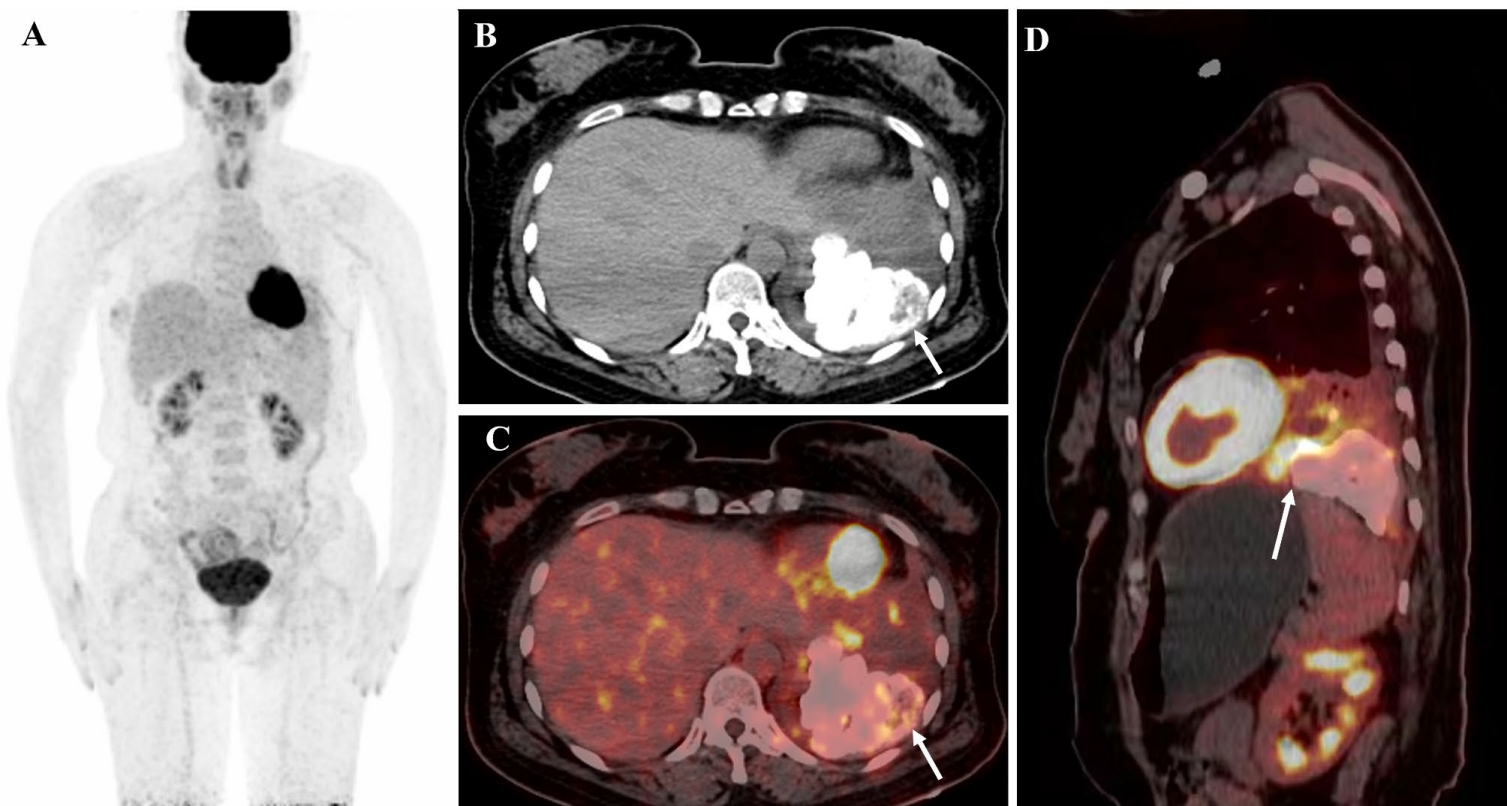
The maximum intensity projection PET Image (A) in the whole body. Transverse chest CT showing a huge tumor with massive calcification (B), and fusion PET/CT (C) images demonstrating a huge tumor with mild high metabolic activity (SUVmax 4.4) and the size of it about 76 mm × 70 mm × 59 mm. Sagittal PET/CT image (D) demonstrating the site of the highest metabolism (SUVmax 8.1)
